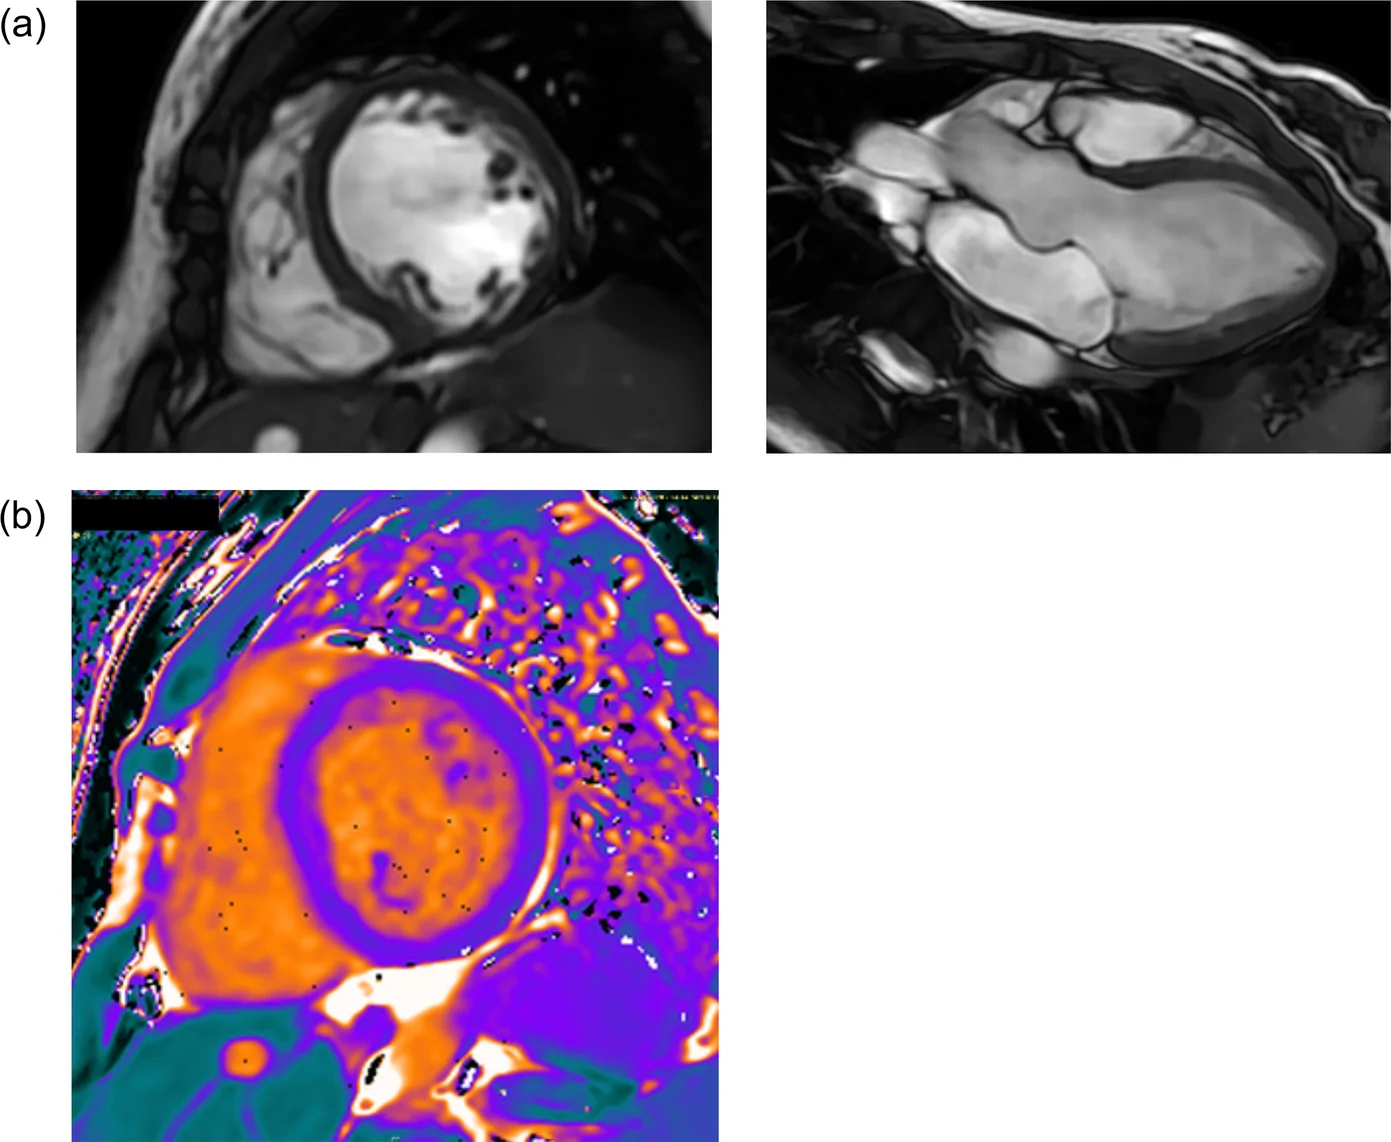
Cardiac MRI findings. (a) Intramyocardial fibrosis. (b) Increased T1 relaxation time (basal septal 1,112 ms).
